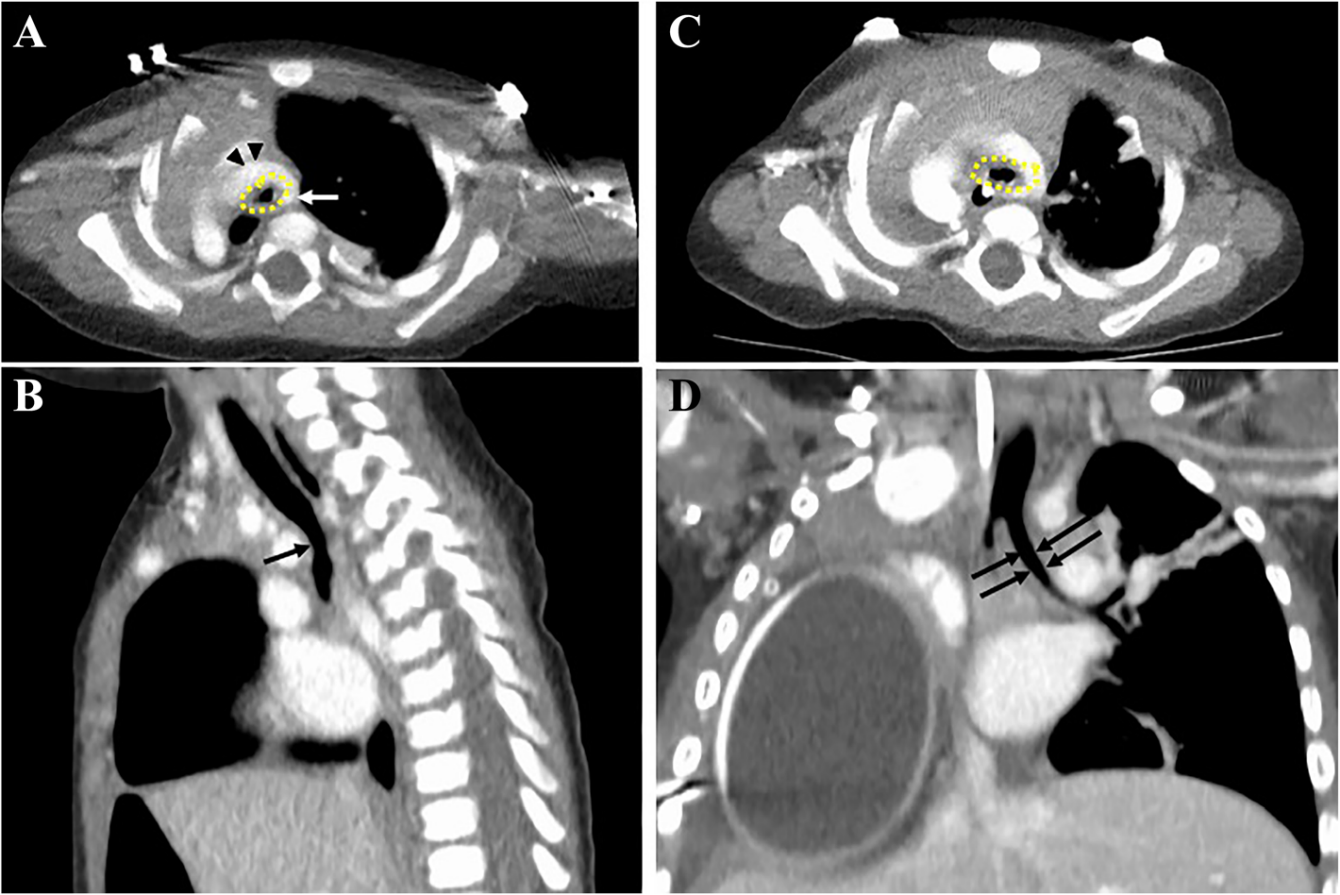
a A CT image shows a functional pseudo-vascular ring formed by the aorta (black arrow heads) and the aberrant right subclavian artery (white arrow) causing tracheal compression (dotted yellow outline). b A sagittal view of a CT image showing tracheomalacia and compression from the pseudo-vascular ring (black arrow). c, d A post-operative CT image after the placement of a tissue expander in the extrapleural space, showing the improvement of the tracheal compression (C: dotted outline and D: black arrows)
